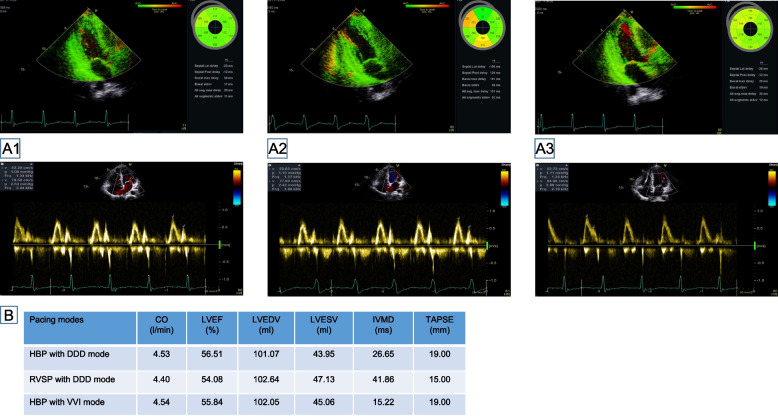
Echocardiography at 1-year follow-up. Echocardiography at 1-year follow-up showed cardiac mechanical synchrony and ventricular systolic function in three different pacing modes. A1: HBP with DDD mode; A2: RVSP with DDD mode; A3: HBP with VVI mode. B: RVSP exhibited a greater intraventricular mechanical delay (IVMD) compared with HBP pacing. HBP with DDD mode showed optimum atrioventricular synchrony and left ventricular systolic function. HBP: His bundle pacing; RVSP: Right ventricular septal pacing; CO: Cardiac output; LVEF: Left ventricular ejection fraction; LVEDV: Left ventricular end-diastolic volume; LVESV: Left ventricular end-systolic volume; APEI: Aortic pre-ejection time; PPEI: Pulmonary pre-ejection time; IVMD: Intraventricular mechanical delay; TAPSE: Tricuspid annular plane systolic excursion
